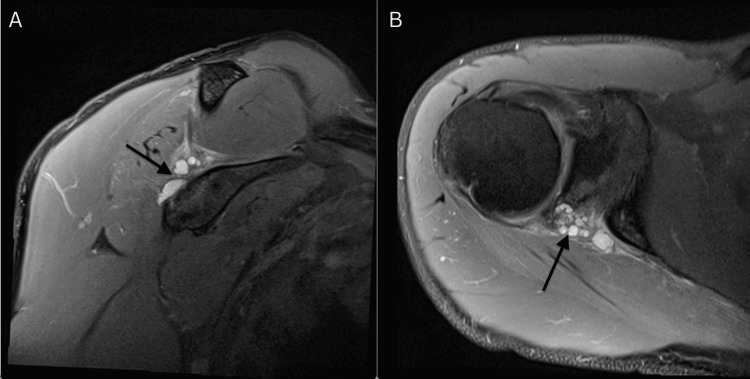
Post-aspiration MRI image: post-aspiration MRI demonstrating the resolution of the paralabral cyst and alleviation of nerve compression (A) Post-aspiration coronal MRI image demonstrating the resolution of the paralabral cyst and alleviation of nerve compression. (B) Post-aspiration axial MRI image demonstrating the resolution of the paralabral cyst and alleviation of nerve compression. Arrow shows a significant decrease in fluid collection.
